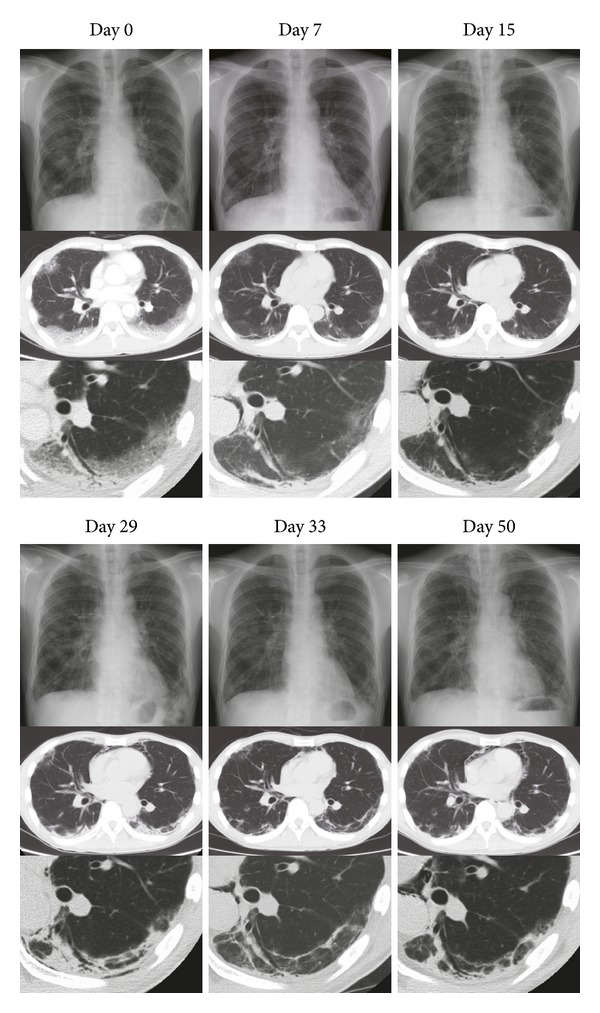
Chest X-rays and computed tomography (CT) scans of the patient with clinically amyopathic dermatomyositis (C-ADM) before and after polymyxin b-immobilized fiber column hemoperfusion (PMX) treatment. Day 0, on admission. Day 7, improvement after initial treatment. Day 15, before relapse. Day 29, on relapse and before PMX treatment. Day 33, improvement after PMX treatment (on days 30 and 31). Day 50, twenty days after PMX treatment.
